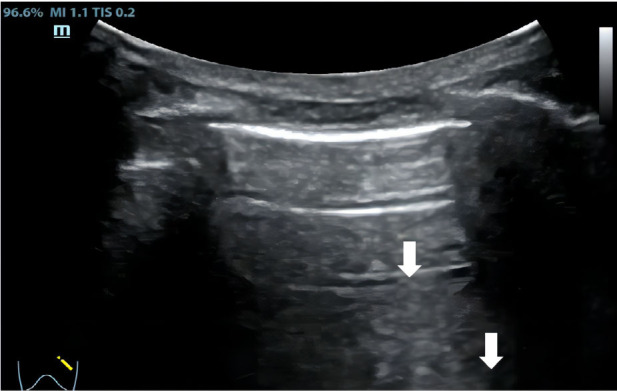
Female aged 63 years old with suspected high-risk pulmonary embolism. In pulmonary ultrasound, the white arrow indicated the A-line. Wedge-shaped solid hypoechogenicity was discovered in subpleural lung tissue.
